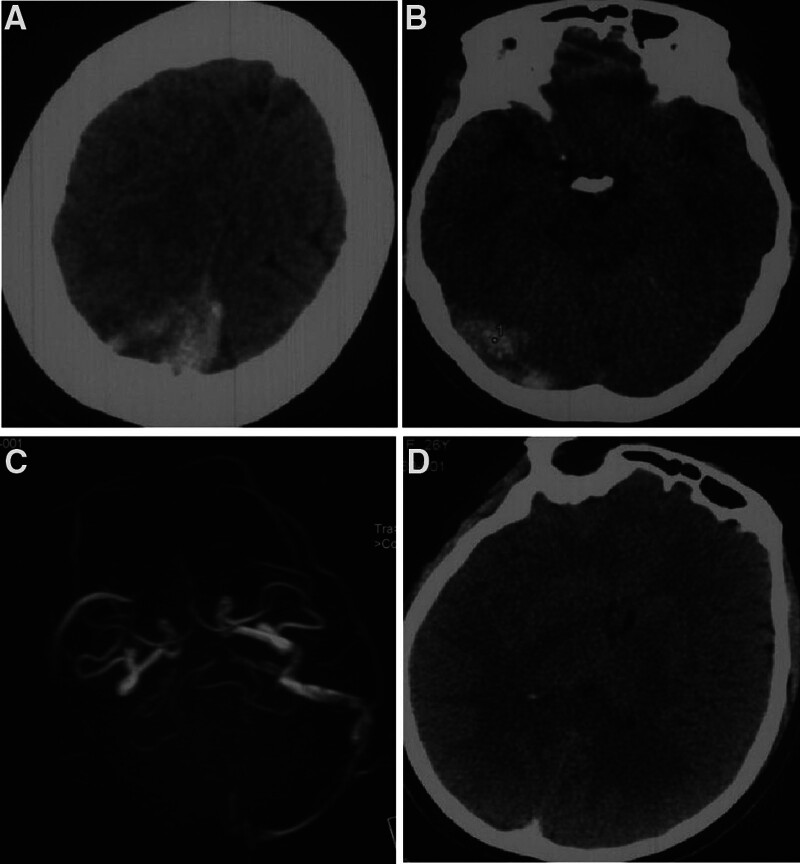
A 27-year-old woman in her early pregnancy had cerebral hemorrhage. (A and B) Non-contrast head computed tomography (CT) demonstrated occipital hemorrhage surrounded by a large hypodense area. (C) Magnetic resonance imaging (MRI) revealed absence of continuous flow within the superior sagittal sinus (SSS), the right transverse sinus (TS), and the right ligmoid sinus. (D) CT scan showed diffuse brain swelling with compressed ventricles.
